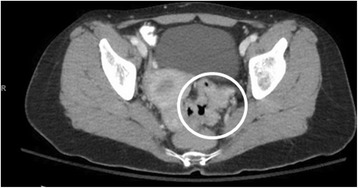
Preoperative CT scan demonstrating a focal area of irregular wall thickening and associated luminal narrowing at the level of the rectosigmoid junction
